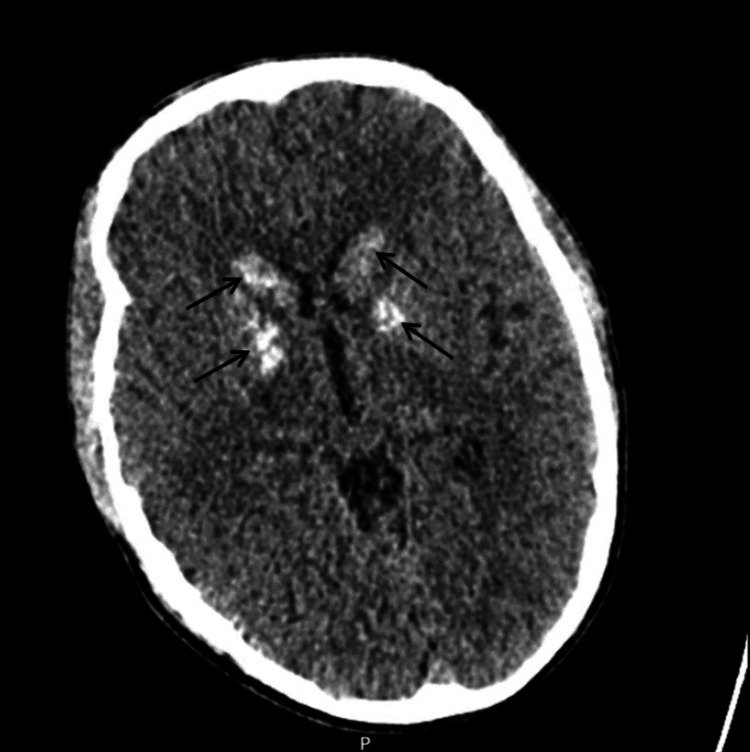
Transverse section through a non-contrast CT head study revealing hyper-attenuation in both basal ganglia (marked out with indicating arrows in the figure)
